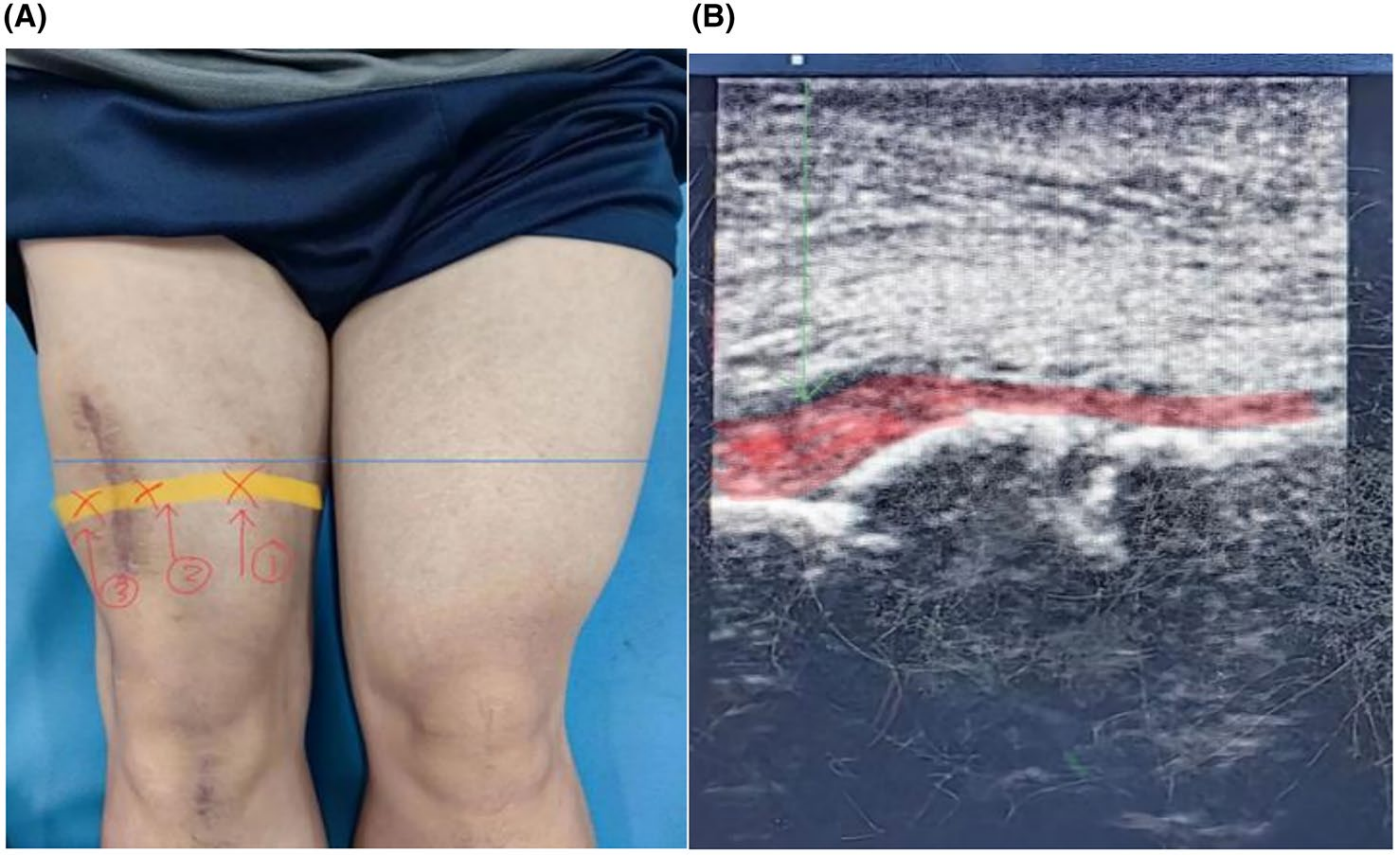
(A and B) Ultrasound‐guided multi‐point injection of PRP along localized markers for treatment.
